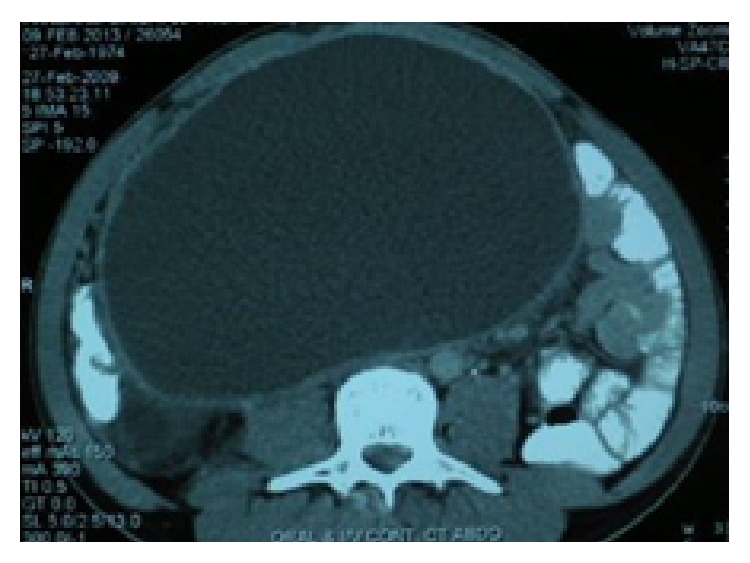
Contrast enhanced CT scan showing retroperitoneal cyst.
